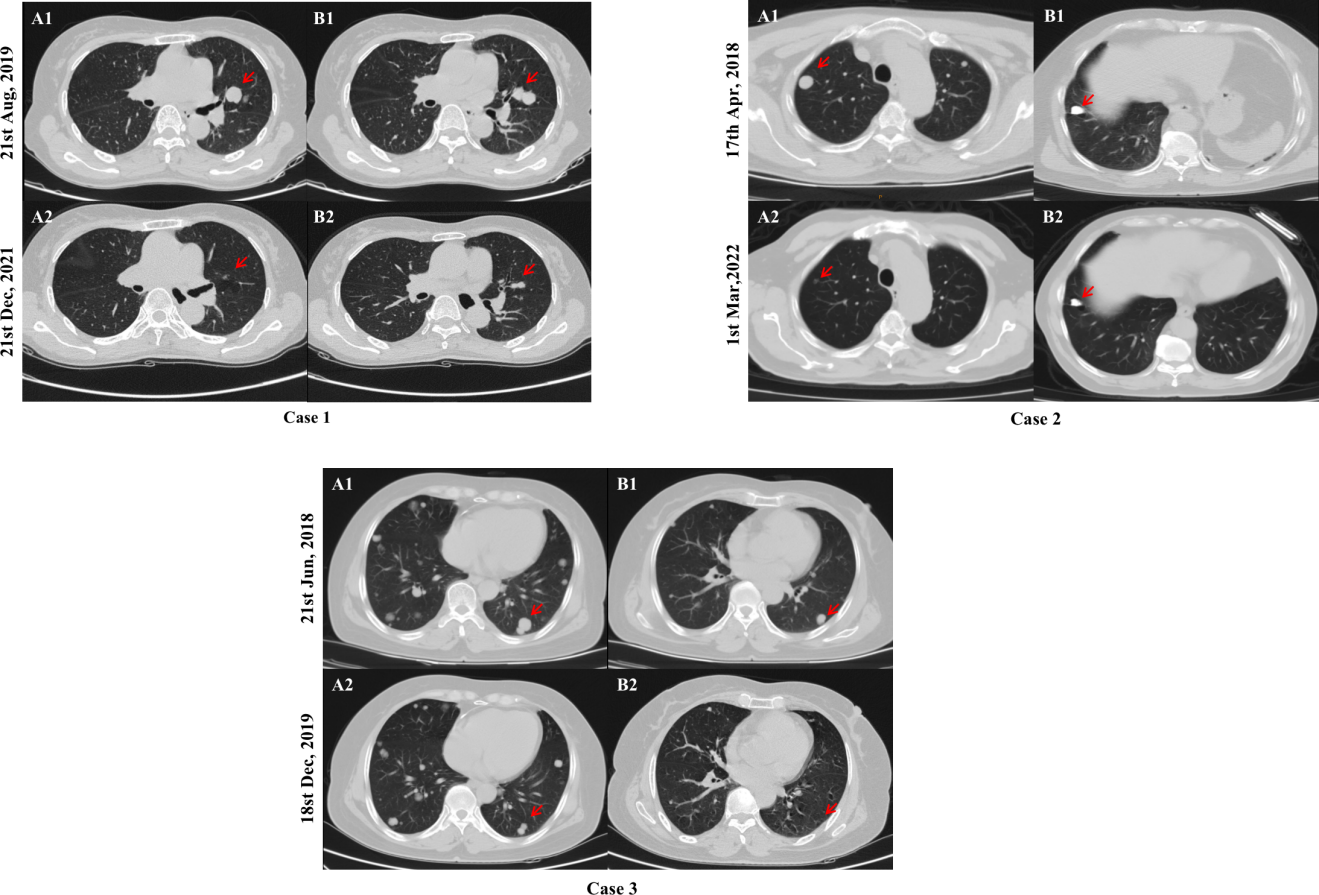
Comparison of chest computed tomography scans of typical cases. After 28, 46, and 17 months of sorafenib treatment, a significant reduction in the size of target lesions was observed in cases 1, 2, and 3, respectively. Chest computed tomography scans at baseline (A1 and B1) and the end of follow-up (A2 and B2) are shown above. The red arrows indicates the location of the target lesions at baseline and the last follow-up.
